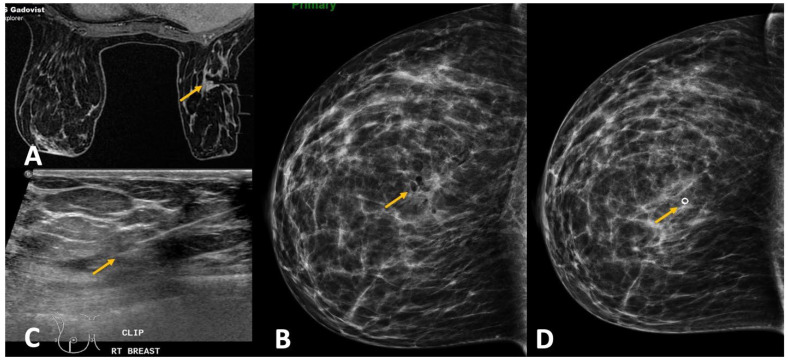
(A). MRI-guided biopsy of right breast for a 7 mm suspicious mass (yellow arrow) was performed to rule out synchronous cancer. The procedure was uneventful. (B). Post-biopsy check mammogram showed absent marker clip and air within the biopsy cavity (yellow arrow). An expedited pathology report was requested and returned as invasive ductal carcinoma. (C). Ultrasound revealed a small hematoma at the biopsy site and hence an ultrasound guided marker clip was deployed (yellow arrow). (D). Repeat check mammogram confirmed the marker clip at the expected location (yellow arrow).
